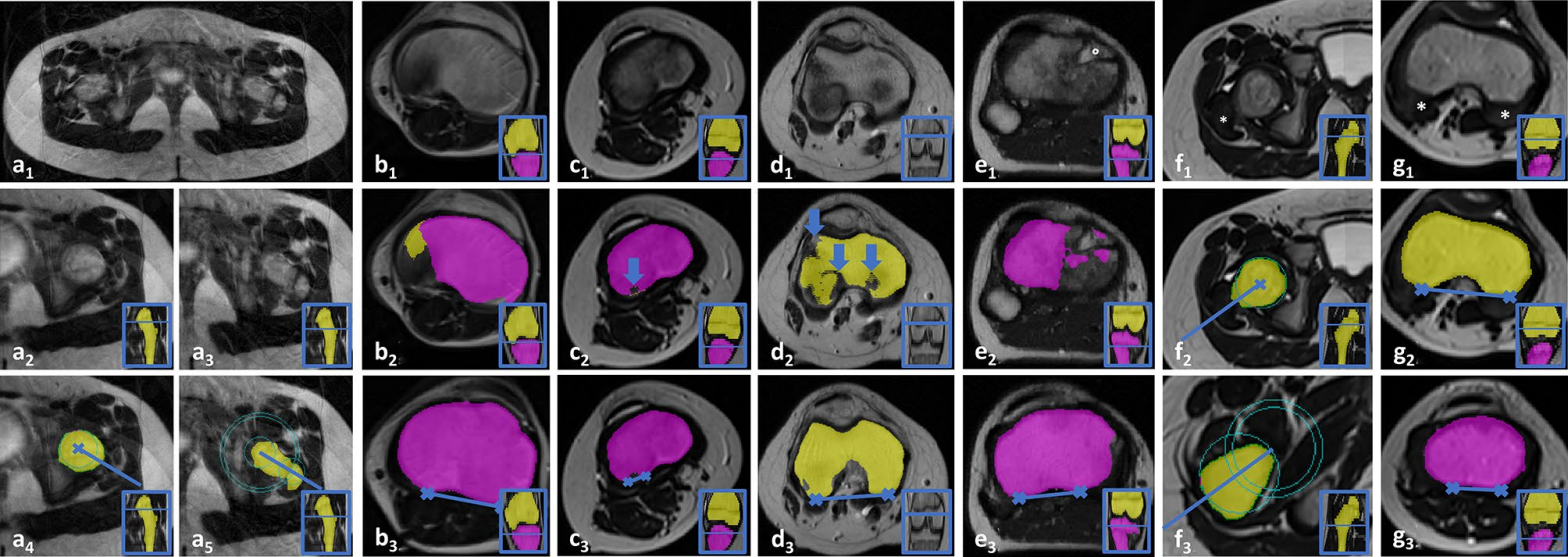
Representative cases that challenged correct segmentation and determination of reference lines. (a) Motion artefacts during the pelvic MRI scan (a_1_) caused smearing of the images at the level of the femoral head (a_2_) and neck (a_3_), erroneous delineation of the femoral head contour (a_4_), and incorrect identification of the femoral neck axis through the greater trochanter (a_5_). (b) Motion artefacts during the MRI scan of the knee (b_1_) created an artificial protrusion of the medial femur (b_2_) that did not negatively affect the reference line (b_3_). (c, d) Prominent and variable epiphyseal plates at the tibia (c) or femur (d) were associated with inaccurate segmentations (block arrows in c_2_, d_2_) that caused misplacement of the reference line (c_3_) or not (d_3_). (e) Post-surgical changes and fat graft interposition at the anteromedial tibia (°) after physiolysis (e_1_) caused inaccurate segmentation (e_2_) but did not negatively affect the reference line (e_3_). (f) At the hip, immature bone characterized by the cartilaginous greater trochanter and femoral neck (* in f_1_) is segmented incorrectly (f_2_) and the femoral neck axis is identified too caudal (f_3_). (g) Similarly, cartilaginous femoral and tibial condyles (* in g_1_) are characzterized by incorrect segmentations and reference lines (g_2_, g_3_). Inset coronal images framed in blue indicate the height of the axial slices. Patient age and gender: 9 years and male (a, c), 11 years and male (b, e), 12 years and male (d), 5 years and male (g).
